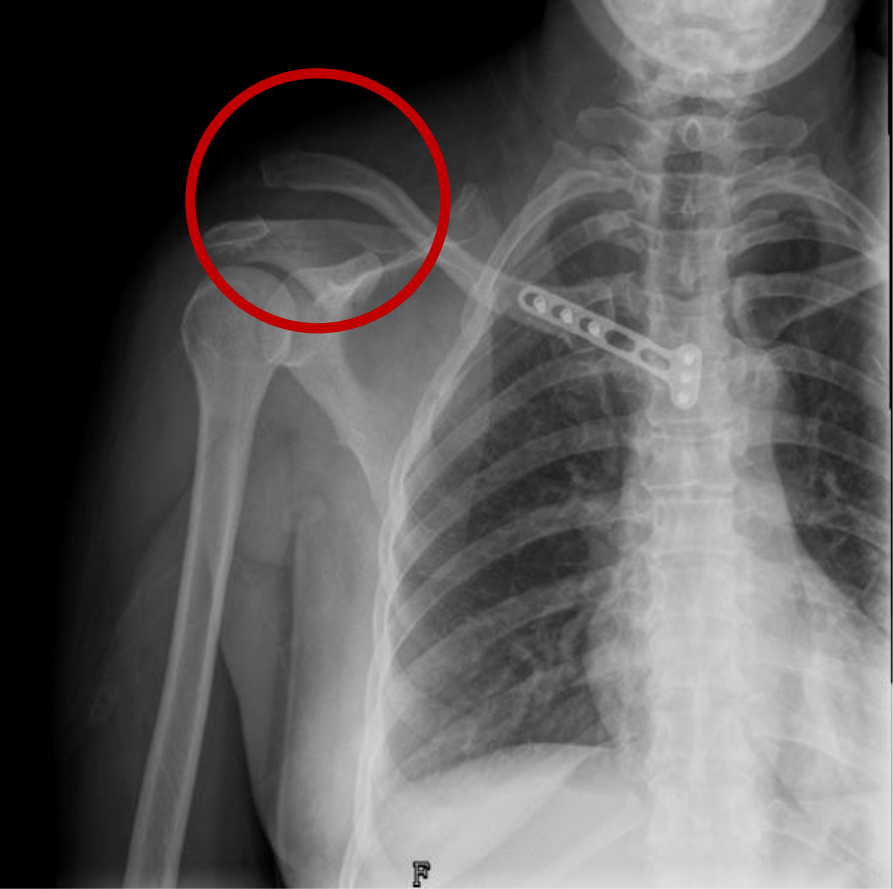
A postoperative x-ray showing right acromioclavicular joint dislocation
